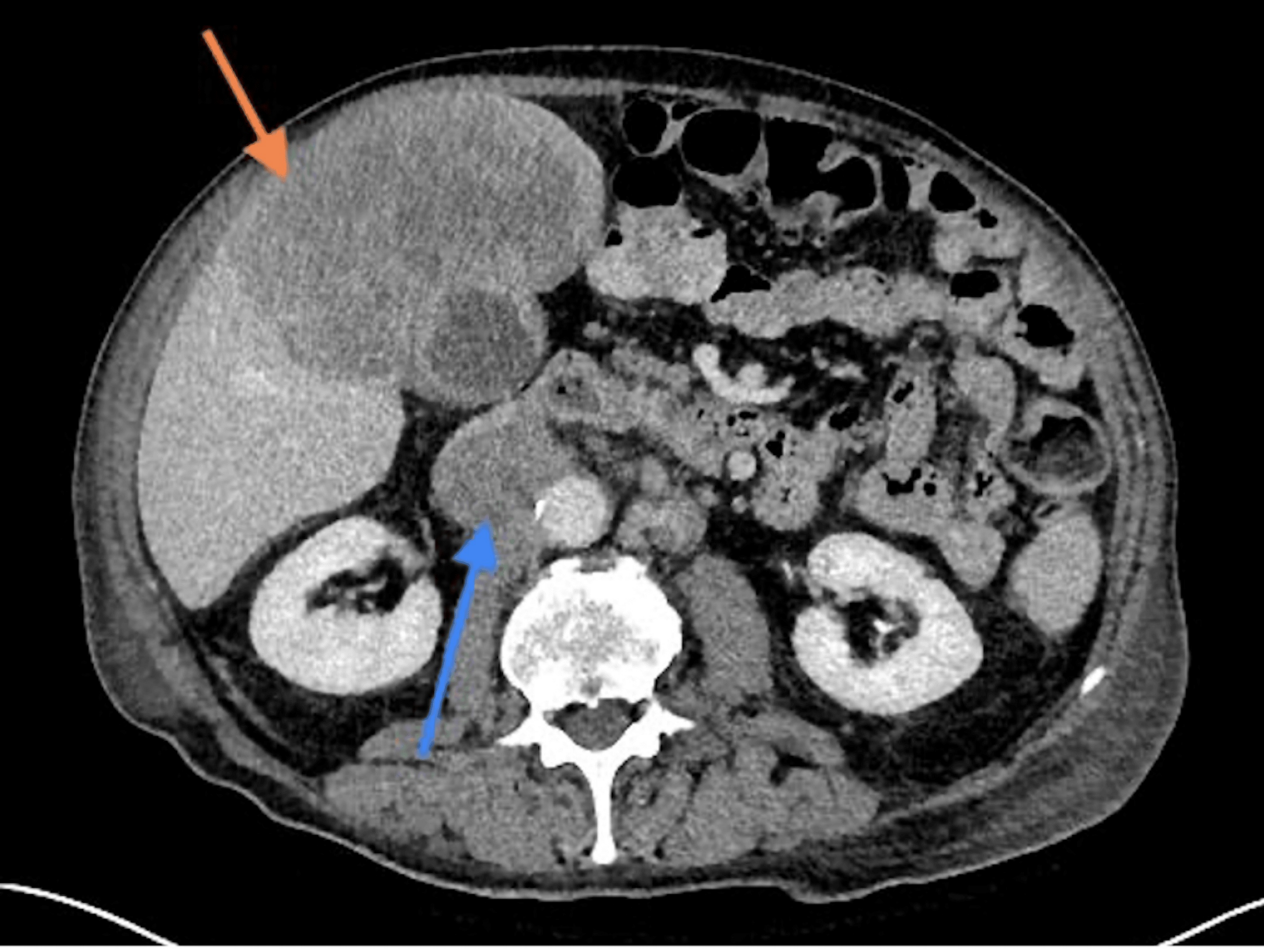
CT abdomen, transverse section. Orange arrow: mass surrounding the gallbladder; Blue arrow: inferior vena cava (IVC) tumor thrombus.
